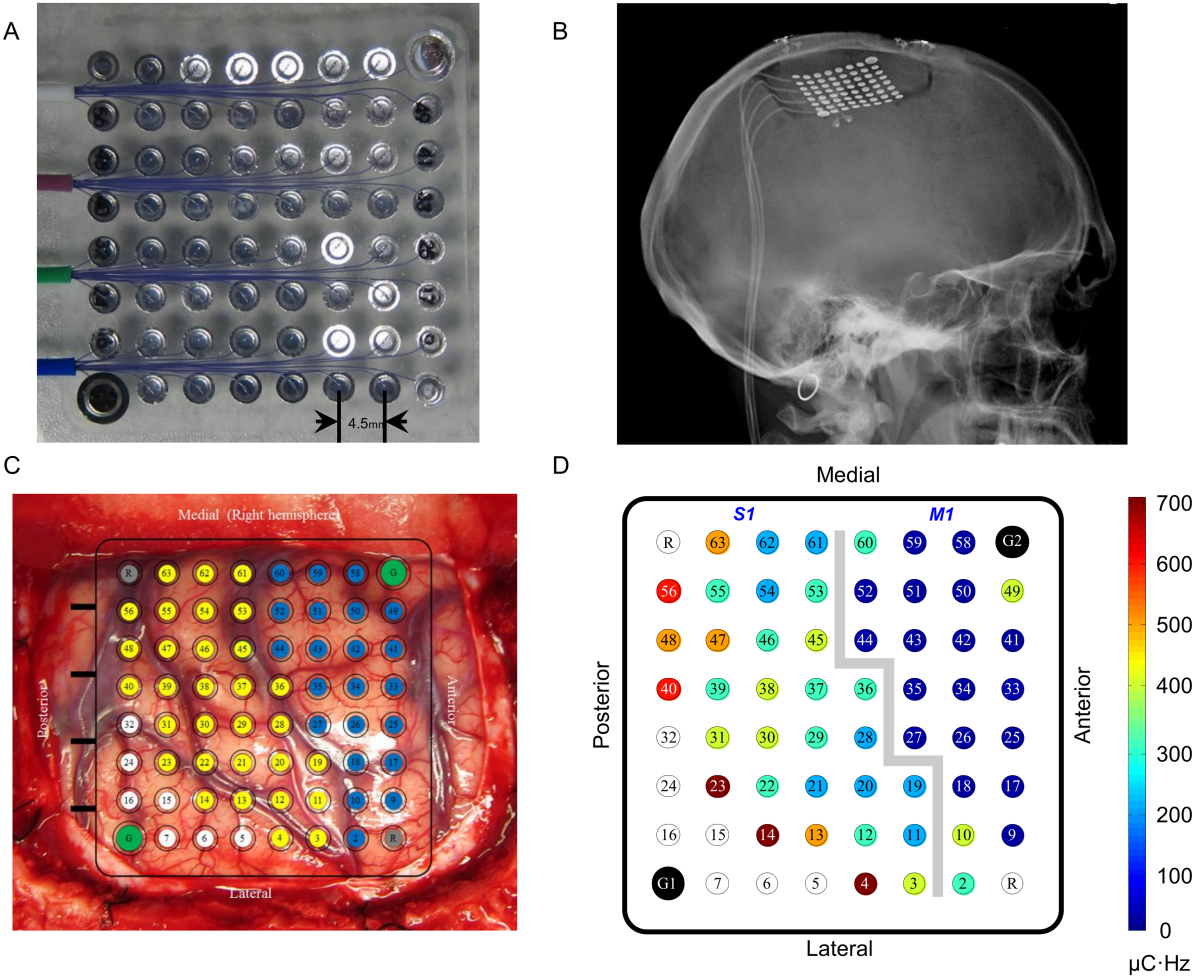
ECoG grid and activation thresholds. *A*: Photograph of the high-density ECoG grid used in this study. The camera is looking at the back of the grid, the non-brain facing surface. The grid was composed of a silicone sheet (4 cm × 4 cm in size, 1 mm thick) with 64 platinum electrodes. The electrodes were 2 mm in diameter and 4.5 mm apart (center-to-center), except for the two ground electrodes that were 3 mm in diameter and 5 mm from the neighboring electrodes (center-to-center). All electrodes were connected to platinum lead wires that formed four 60-cm long leads. *B*: Post-operative skull radiograph showing the ECoG grid. *C*: Grid location on brain surface. The blue electrodes are above the motor cortex (M1). The yellow electrodes are above the somatosensory cortex (S1). The green and grey electrodes are dura-facing ground and reference electrodes, respectively. The white electrodes did not generate a response to any cortical stimulation attempted within the safety limits of our study (less than 57 μC/cm^2^ per phase). *D*: Activation thresholds. G1 and G2 (black electrodes) were the return electrodes for Electrodes 1–32 and Electrodes 33–64, respectively. The threshold values are presented in terms of charge exchange per second (μC•Hz) required to elicit a response. The gray line separates the electrodes above the motor and somatosensory cortices. The stimulation pulse amplitude, width, and frequency ranged from 1 to 7 mA, 50 to 400 μs, and 50 to 500 Hz, respectively. The color bar represents the range of the charge exchange.
